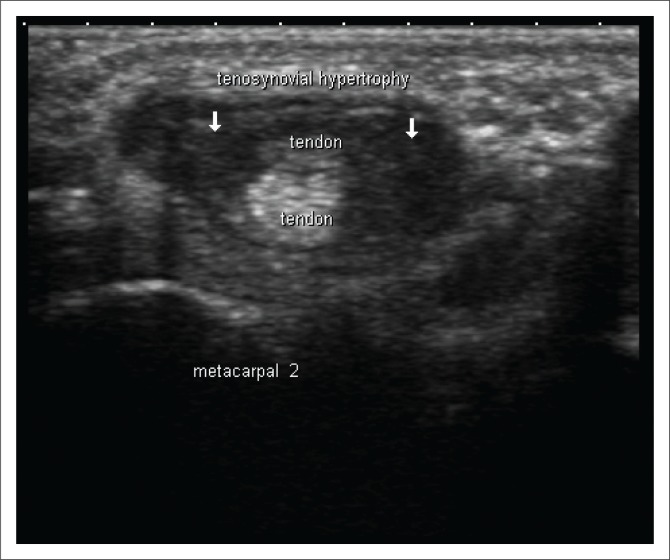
Transverse ultrasound image at the level of the second metacarpal demonstrating tenosynovitis of the extensor tendons of the hand.
